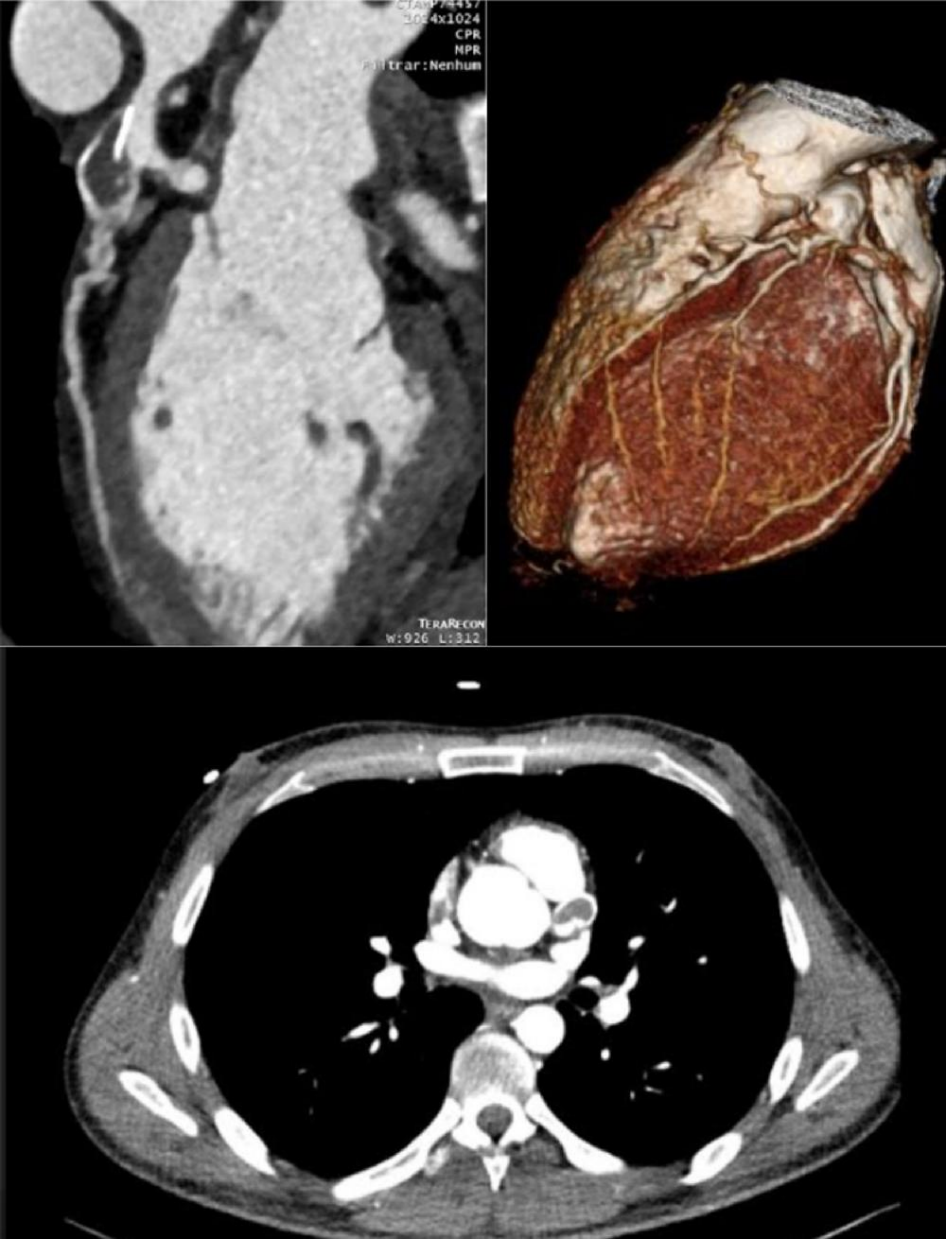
Computed tomography angiography—aneurysm involving the distal left main trunk and the ostial portion of the left anterior descending artery, with thrombosis causing subocclusive stenosis. Two sequential aneurysms are visible in the left circumflex artery, displaying parietal calcification without significant stenosis. The mid-segment of the right coronary artery reveals an ectatic segment with mural thrombosis, remaining patent with anterograde filling through collateral vessels.
